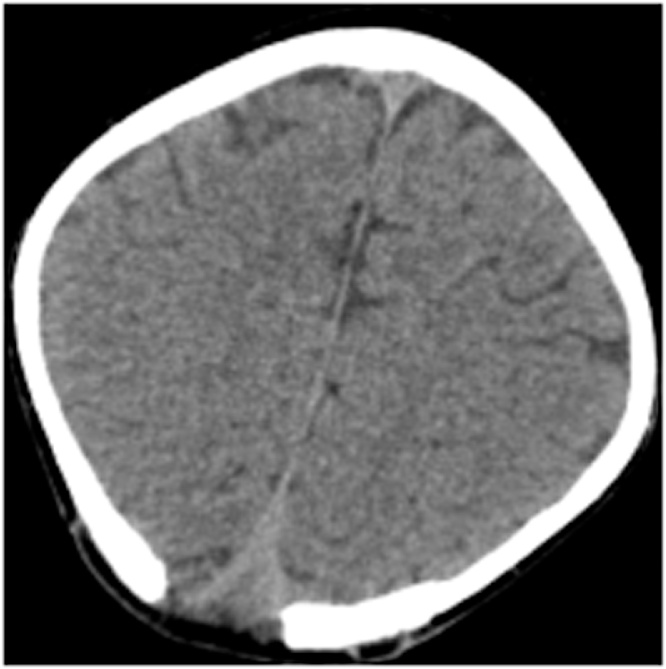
At a one-month postoperative follow-up, CT scan shows no remnant mass.
